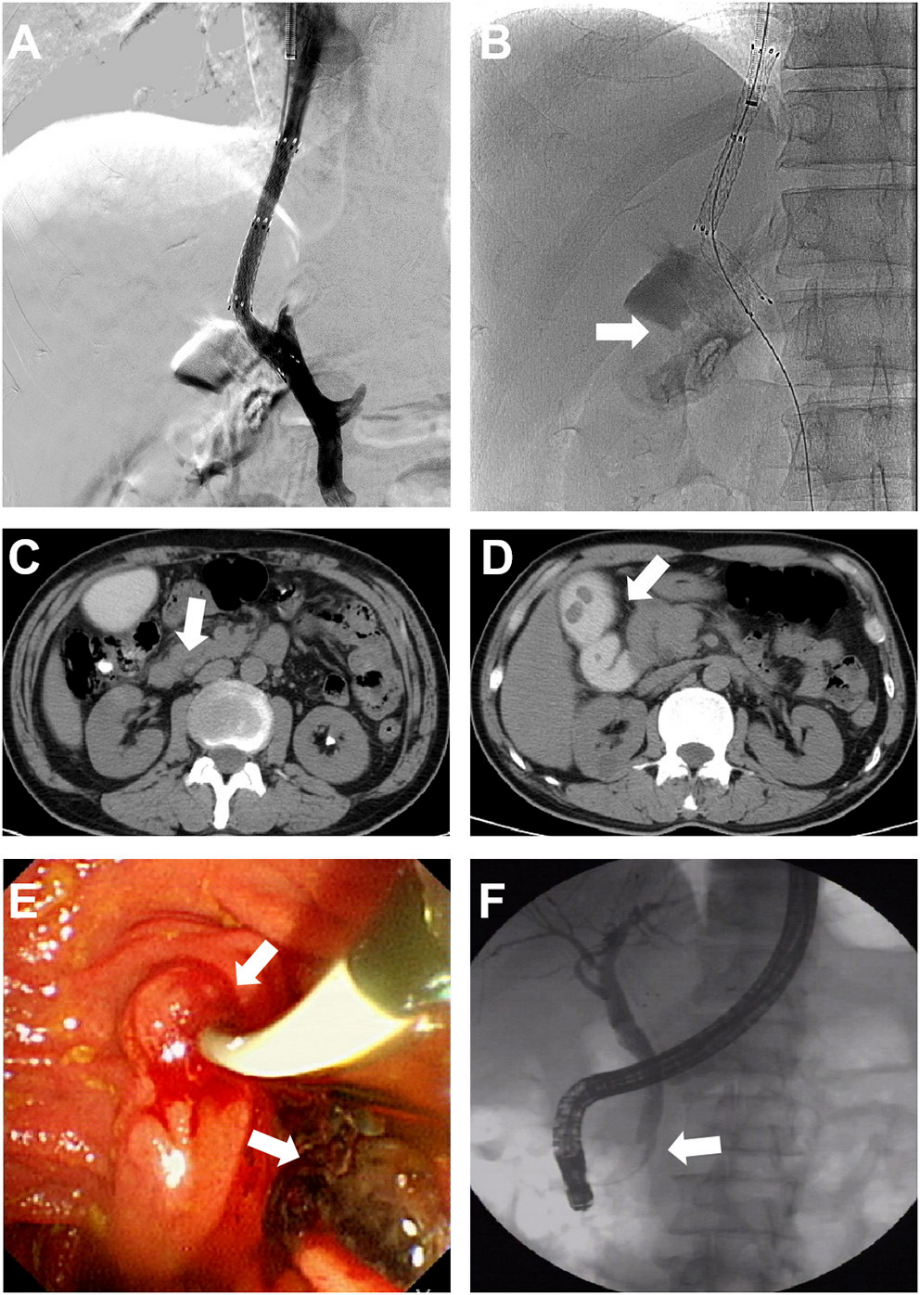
Characteristics and management of hemobilia. (A,B) Bile duct was punctured during the procedure and contrast medium retention was found in the gallbladder. (C,D) Serum bilirubin elevated gradually after TIPS. CT showed bile duct dilatation, retention of contrast medium in the gallbladder, and high-density shadow of the common bile duct. (E,F) Endoscopic retrograde cholangiography (ERCP) showing a filling defect in the common bile duct, and the bleeding clot was cleared. The nasobiliary duct was retained for drainage.
